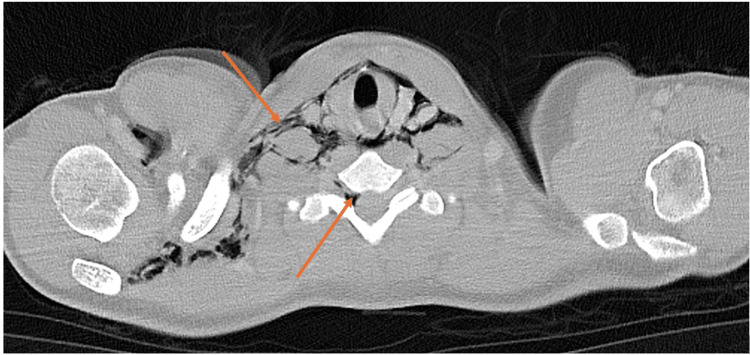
CT showing large volume pneumomediastinum with extensive surgical emphysema of the neck and right axilla/chest wall CT: computed tomography
